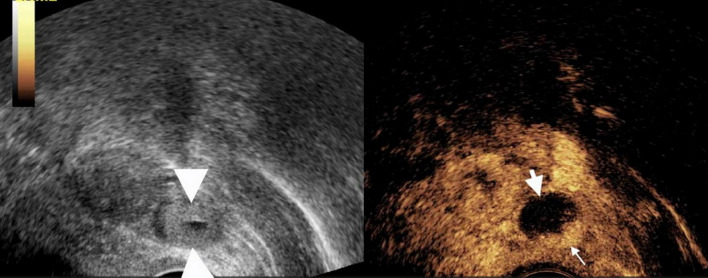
Transvaginal contrast enhanced ultrasound (CEUS) showed the cross section of the fallopian tube. The patient was 34 years old and came to the hospital due to abdominal pain, amenorrhea, and vaginal bleeding. The blood HCG level was 2165 mIU/mL, and the time interval between the CEUS and the measurement of blood HCG was less than 24 hours. A ring-like slightly high echoic structure (bagel sign) measuring 1.7cm × 1.4cm was visible in the left adnexal region, with a small area of anechoic in the center (left figure). CEUS imaging showed that, some parts of the high echoic ring-like structure enhanced, while other parts were not enhanced (right figure). The thick arrow indicated the dilated fallopian tube, and the thin arrow indicated the enhanced villous tissue. The villous tissue size visible during the operation was 0.5cm × 0.5cm, less than the size measured by ultrasound (0.8cm x 1.0cm).The enhancement pattern of this patient was non-annular enhancement. This patient was confirmed by surgery to have a pregnancy in the ampulla of the left fallopian tube
